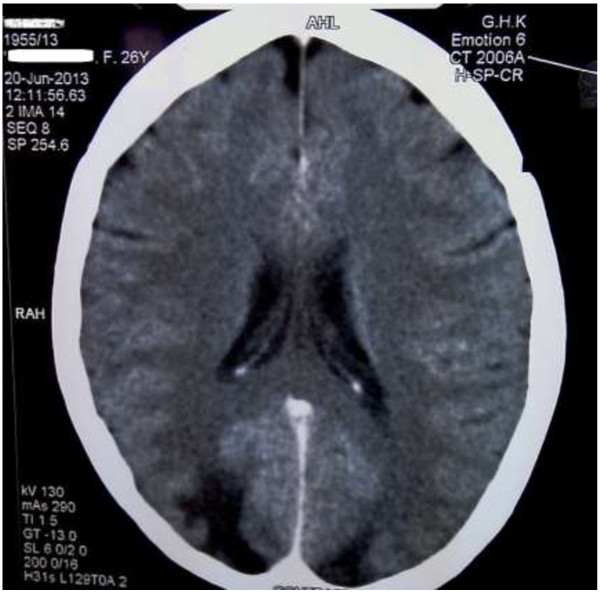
CT brain: Computed tomography scan of the brain taken on preliminary presentation showing bilateral occipital lobe hypodensity and oedema.
